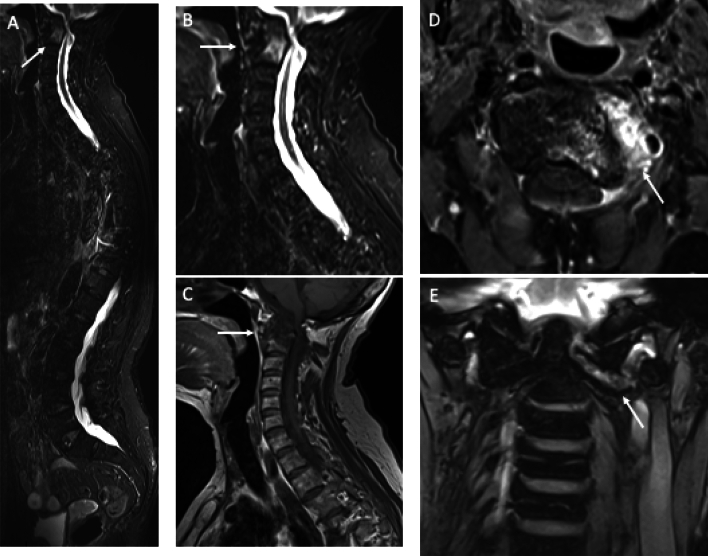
WB-MRI in a 73-year-old woman with atlantoaxial rotatory subluxation due to rheumatoid arthritis (RA). STIR images in sagittal (a and b) and T1-weighted in sagittal (c) planes show that the posterior cortex of the anterior tubercle of C1 and the anterior cortex of the odontoid process are spaced and not parallel. Ax T1 + C (d) show intense synovitis. Cor T2 dedicated (e) also show spacing
